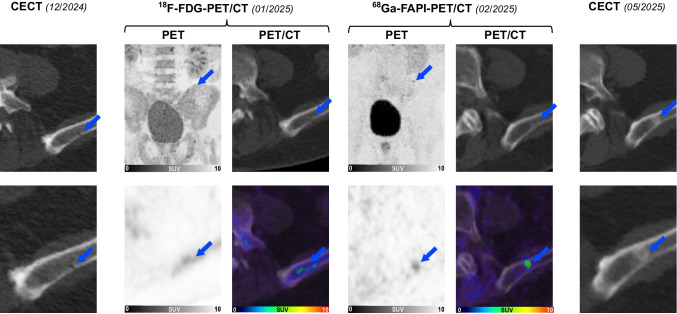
Exemplary case of a bone lesion which was detectable in ^68^Ga-FAPI- but not in ^18^F-FDG-PET (blue arrow). In a previous contrast enhanced CT (CECT) a small osteolysis is already visible in the left ilium (same patient as in 2B). 6 month later, after chemotherapy, blastic demarcation of an initially lytic lesion is evident in a CECT at the site where the ^68^Ga-FAPI-PET/CT previously showed circular enhancement, consistent with response to treatment of an osseous metastasis
